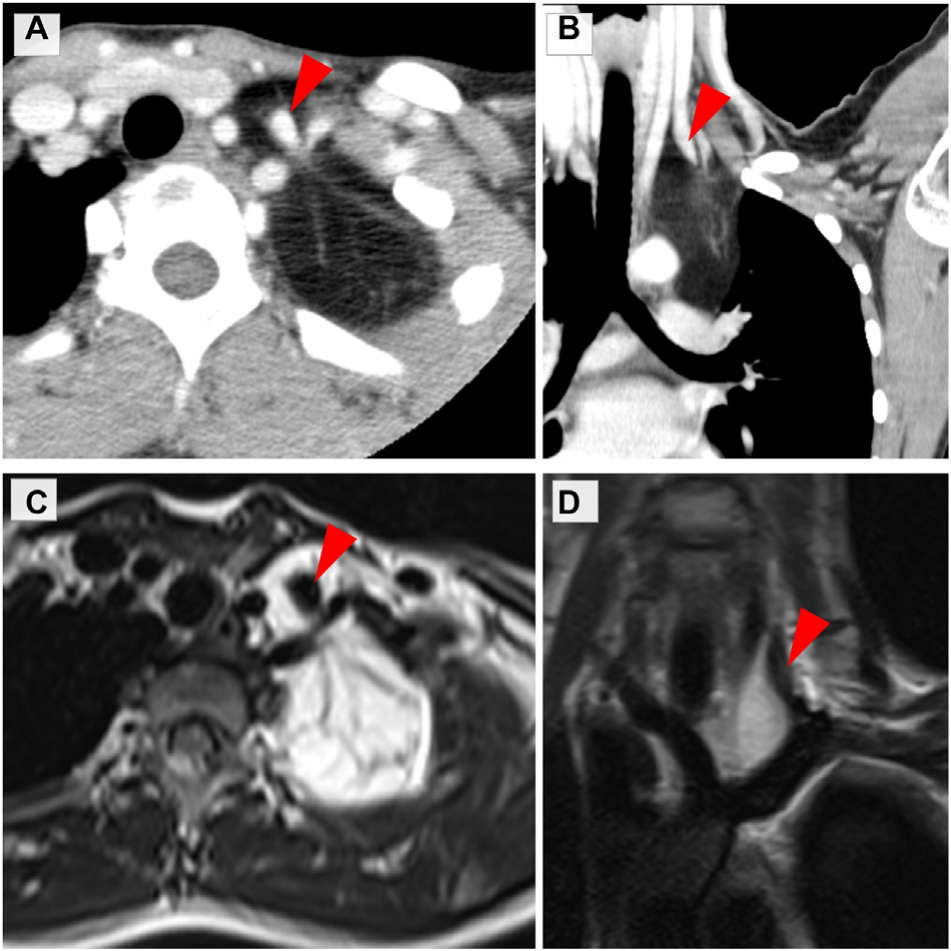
(A, B) Chest computed tomography and (C, D) magnetic resonance imaging revealed a left superior mediastinal lipoid tumor extending to the neck and circumferentially surrounding the internal jugular vein (arrowhead).
